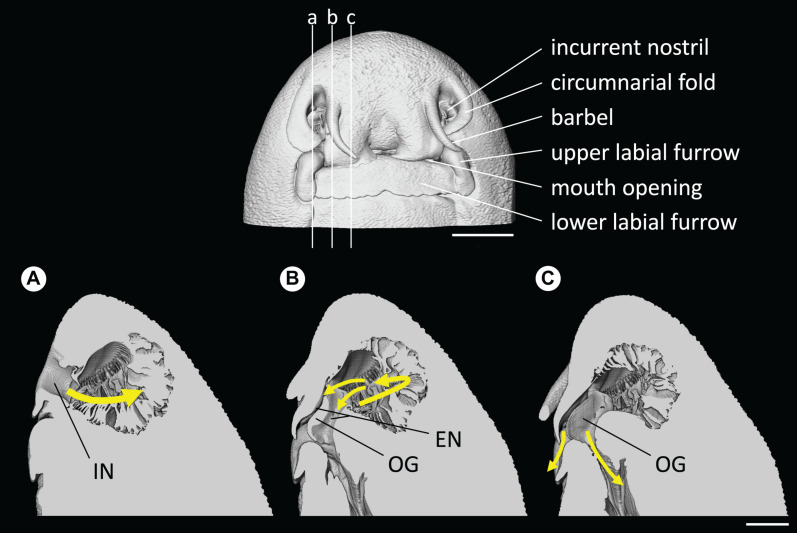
Head and nostril morphology of *Chiloscyllium punctatum*, presented from the volume data (CT). (Top) the vertical lines indicate the position of sagittal slices presented in panels (A–C). (Bottom) main water flow pathway in the olfactory cavity, represented by gradient, colored arrows (a–c). IN, incurrent nostril; EN, excurrent nostril; OG, oronasal groove. Scale bars = 5 mm.
